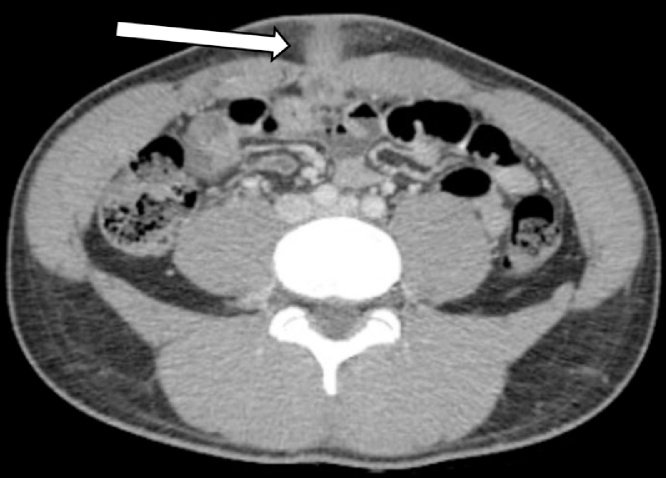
Axial CT abdomen showing urachal remnant extending through the ventral abdominal wall with associated inflammatory infiltrate and central lucency suggestive of abscess.
